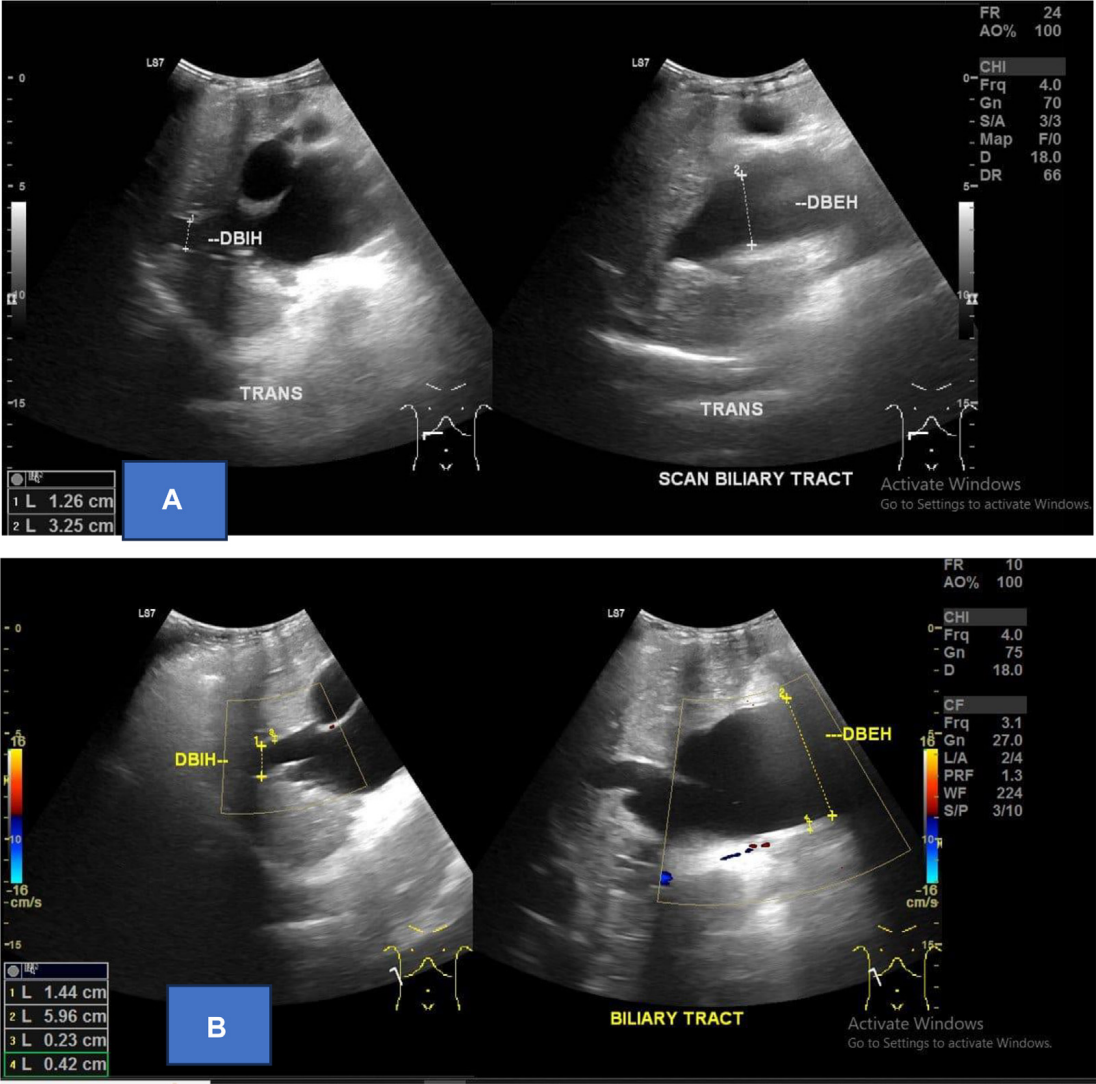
Intrahepatal and extrahepatal ductus US, patients with Choledochal Cyst type IV (A) Ultrasound showed dilatation of intrahepatal and extrahepatal ductus, (B) doppler showed without vascularization.
